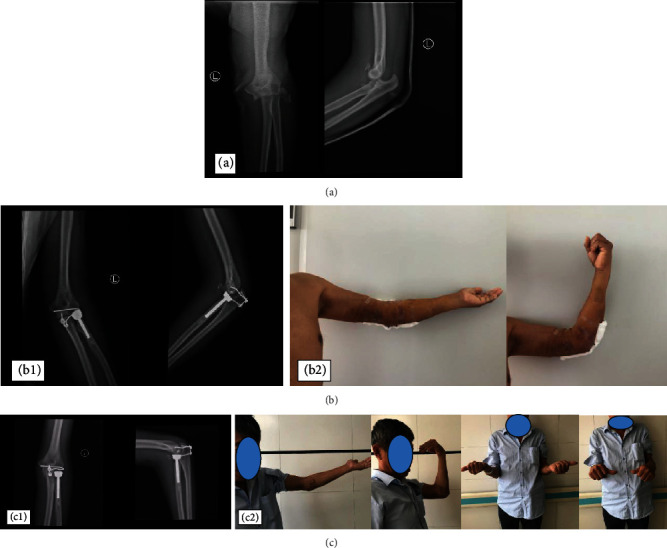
(a) X-ray films of the left elbow show dislocation of the left elbow, comminuted fracture of the radial head, and fracture of the coronoid process. (b-1) X-ray films five days after placement of the device show that the left dislocated elbow has been reduced, the radial head has been replaced, and the device has been placed. (b-2) Active flexion and extension five days after placement. (c-1) X-ray films two months after placement show that the elbow joint alignment is normal, and the device has not become loose, fallen off, or broken. (c-2) Elbow extension function, flexion function, forearm supination function, and forearm pronation function two months after placement.
